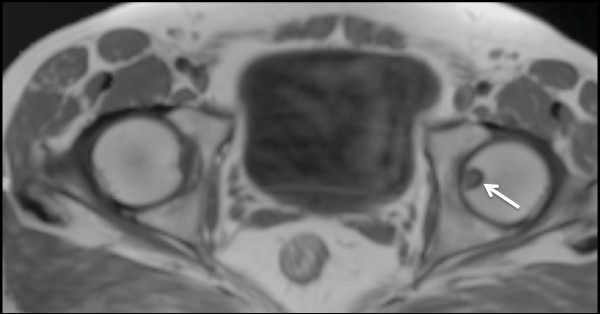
T1-weighted axial MRI showing a corresponding hypointense lesion (white arrow).
